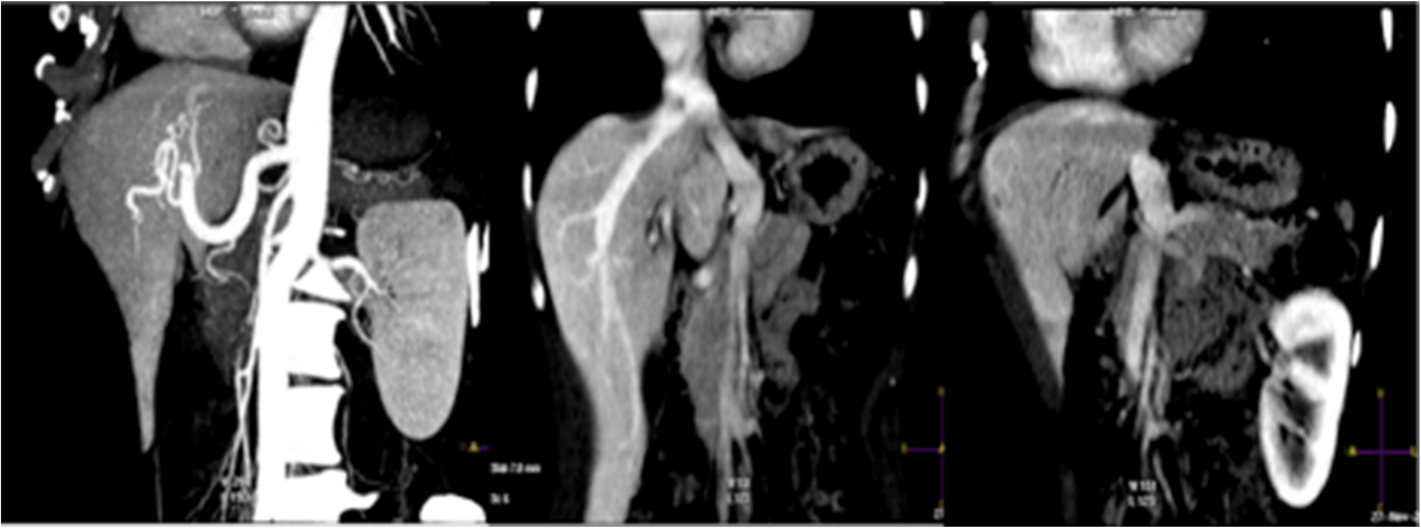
CT coronal planes in arterial and portal phases on case 2 (Type 2-side-to side). Note: The abdominal aorta with an increased caliber of the hepatic artery and its intrahepatic branches hypertrophied. Confluence of the middle hepatic vein and the extra hepatic portal vein to the inferior vena cava
